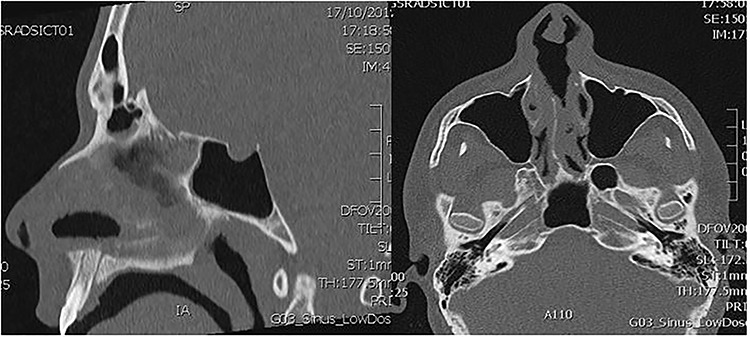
Sagittal and transverse computed tomography section through the nasal septum showing a nasal septum perforation.
